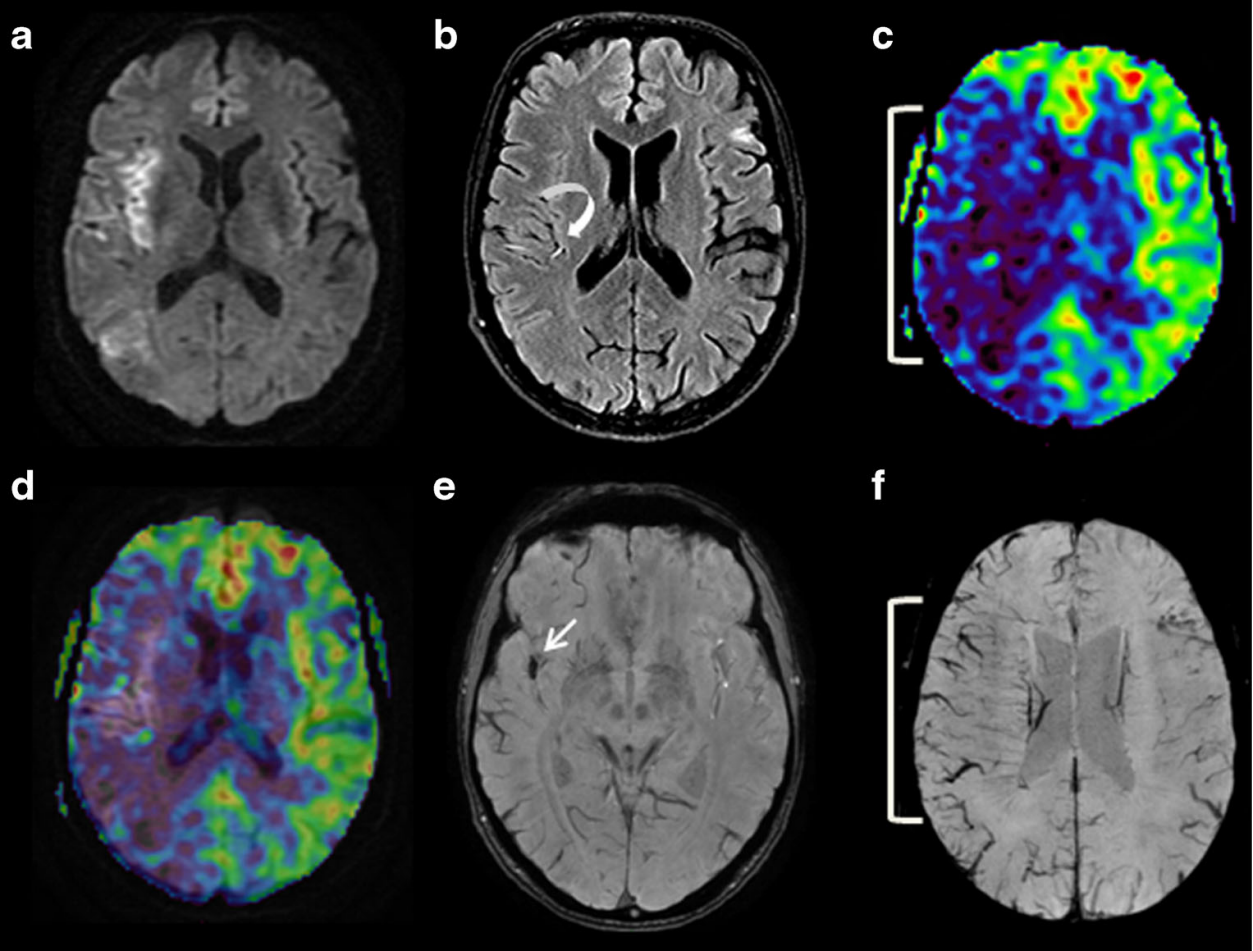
A 76-year-old patient seen in an emergency setting for right brachiofacial motor deficit 3 h after symptom onset. DWI (a), FLAIR (b), ASL (c), ASL/DWI fusion (d), and SWI (e and f) sequences in the axial plane. a Acute right superficial sylvian infarction. b The FLAIR sequence fails to visualise any infarct zone. Slow circulation in the cortical branch of the right middle cerebral artery, hypersignal (*curved arrow*). c Blue zone (*white parentheses*) visualises a wide right sylvian zone of hypoperfusion. d Mismatch: DWI hypersignal and ASL hypoperfusion. e Susceptibility vessel sign in the M2 segment of the right middle cerebral artery, thrombus. f Right sylvian (*white parentheses*) brush sign; the extension is the same as the hypoperfusion zone visible on the ASL sequence (image c)
